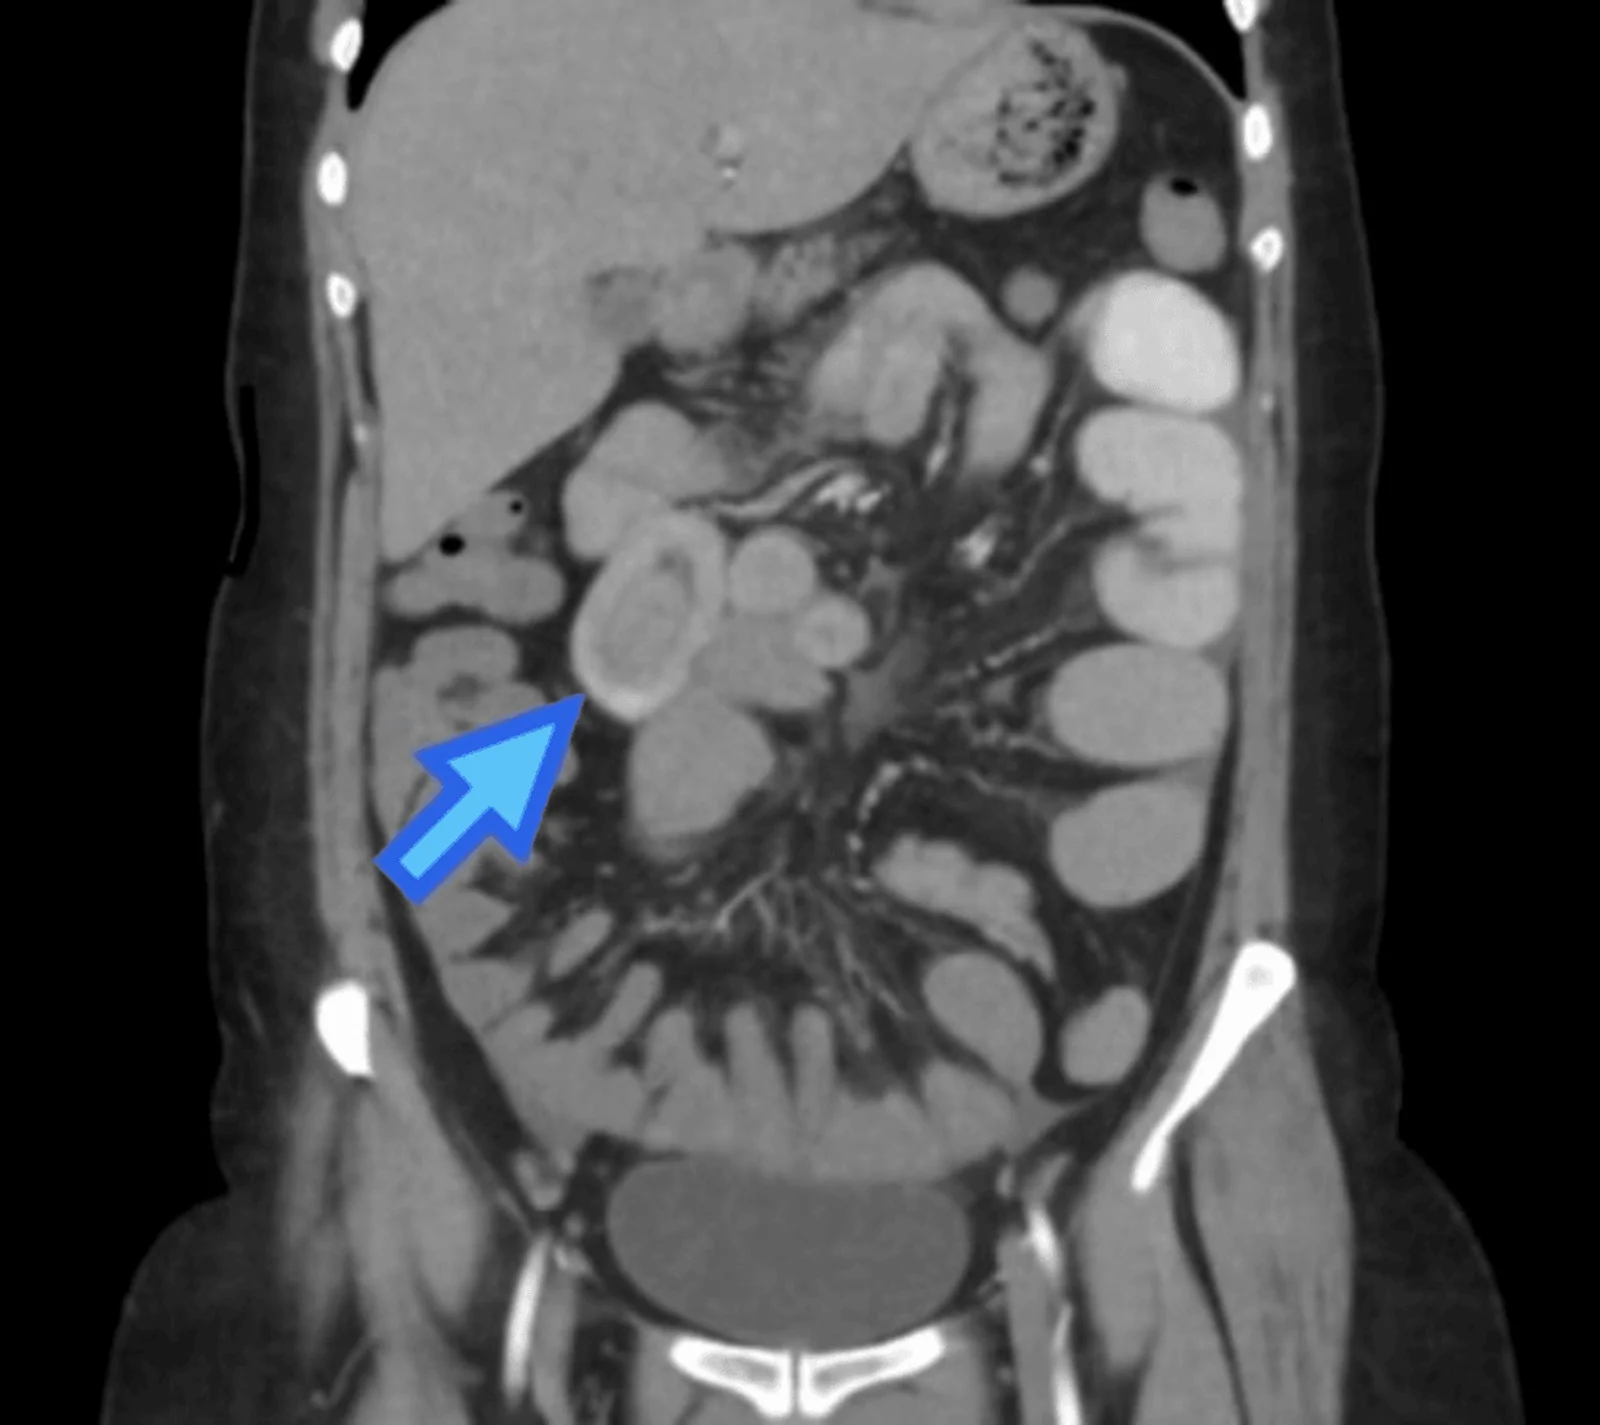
Coronal Contrast-Enhanced CT of the Abdomen A coronal view from the contrast-enhanced CT scan demonstrates the “target sign” or telescoping appearance (arrow) in the distal jejunum. CT: computed tomography
